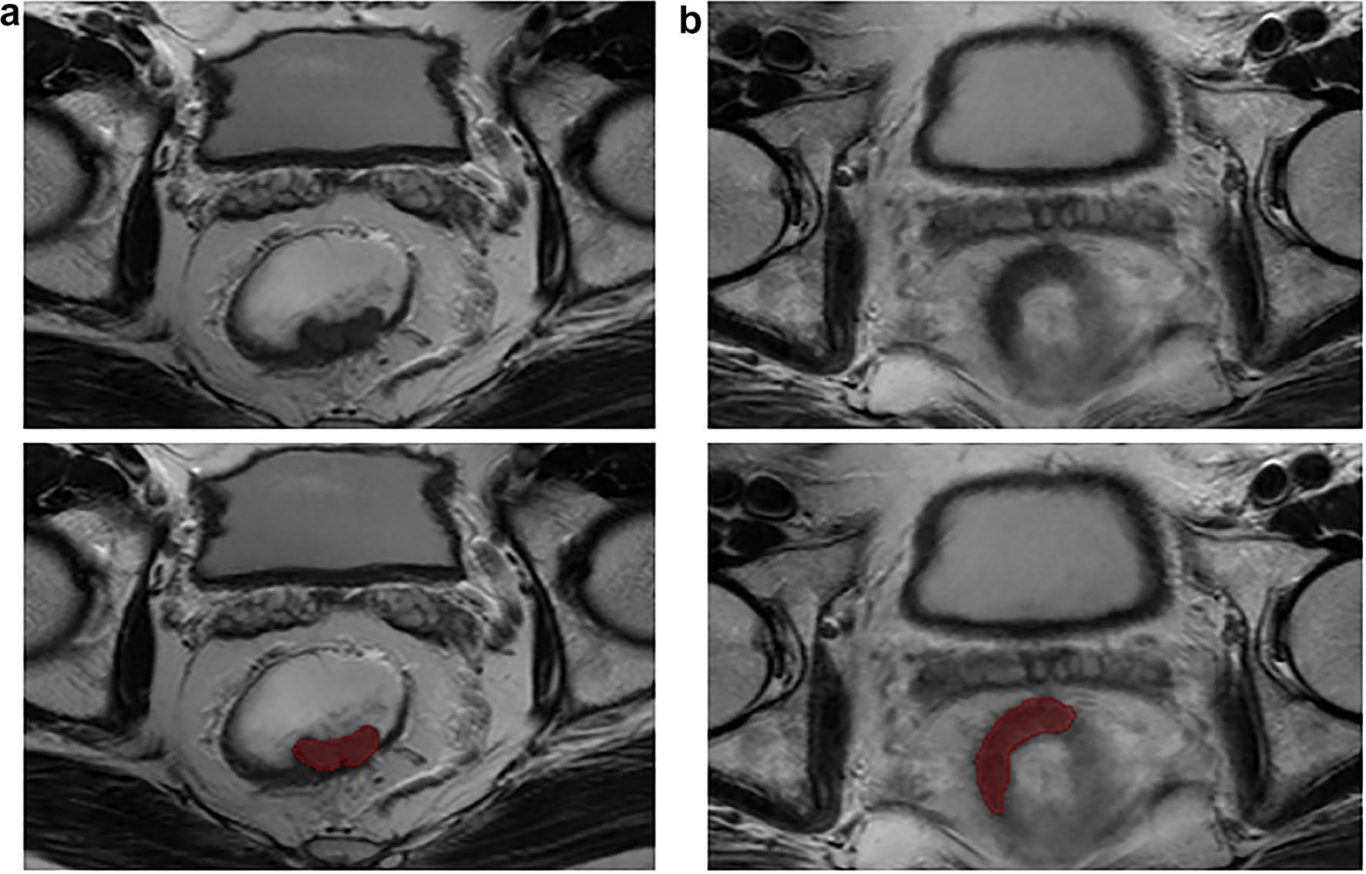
Tumor segmentation on rectal MRI. a A 49-year-old man with early-stage rectal cancer. b A 73-year-old man with LARC
